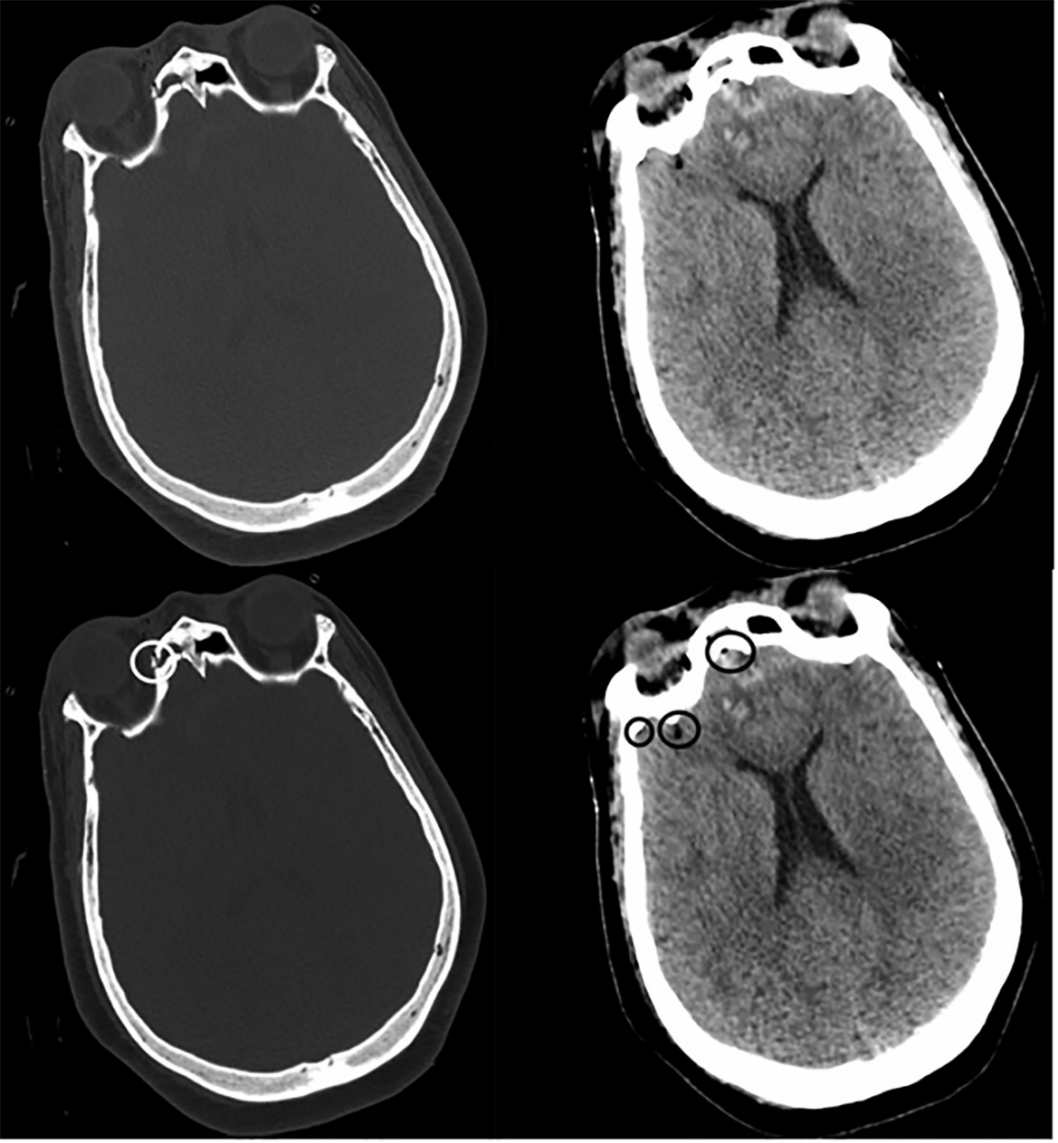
Computed tomography brain without contrast at initial presentation of a
